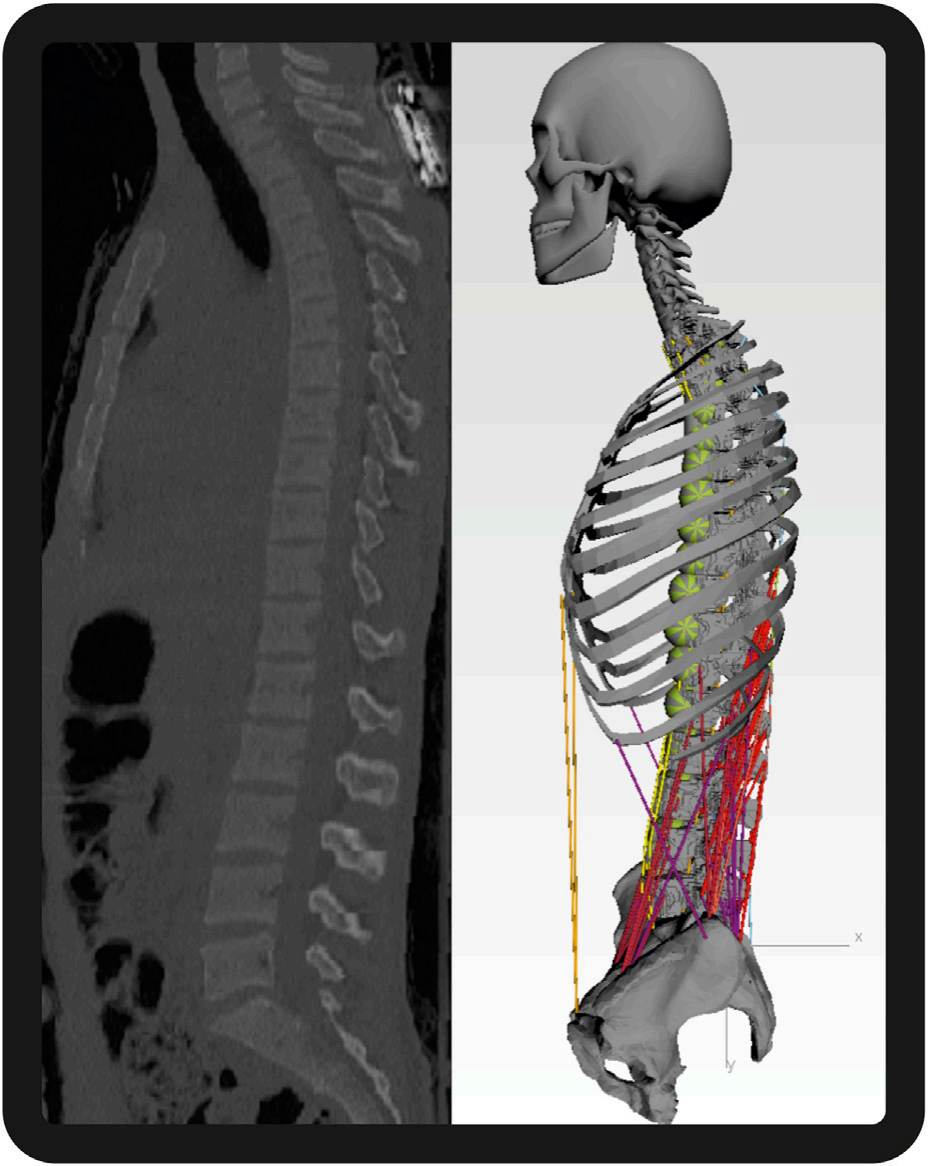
Sagittal CT images of one subject with the respective MBS model. Segment masses for soft tissues are visualized by the green spheres. For the sake of clarity, the dummy bodies for the arms consisting of two scaled cylinders centrally attached to T3 are not shown here [adapted from Lerchl et al. (2022)].
